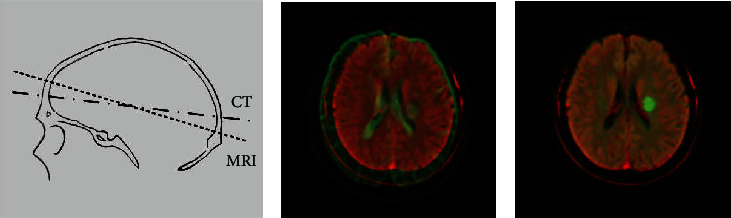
Image registration. (a) DWI was adjusted by multiple planner reconstruction to obtain a consistent angle with CT. Dotted line denoted MRI and point solid line denoted CT. (b) CT and DWI were put together to achieve coarse registration. (c) Fine registration was performed by a series of affine transformation including translation, rotation, and scaling.
